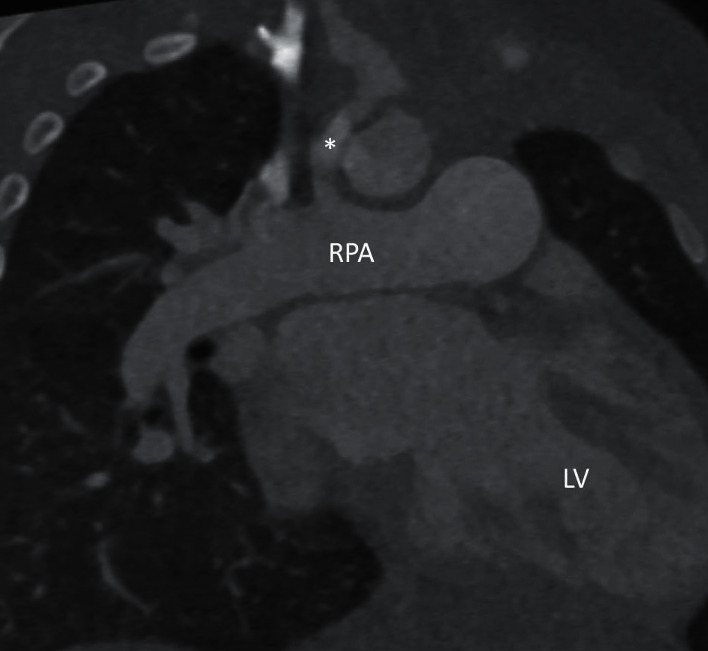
An oblique coronal image from contrast enhanced cardiac CT showing a right modified Blalock-Taussig shunt (^*∗*^), dilated right pulmonary artery (RPA), left atrium, and LV. Note the abnormal LV trabeculations along the LV inferior, lateral and apical walls.
